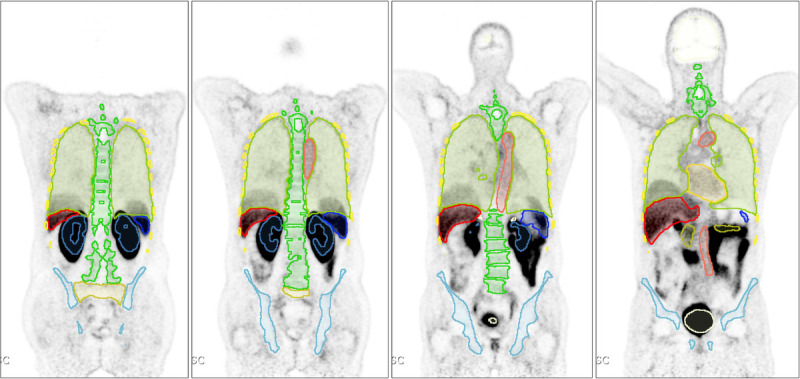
Coronal slices showing representative cases presenting with mismatch between CT-based segmentation and PET images. Please note the chest/abdomen interval organs.
